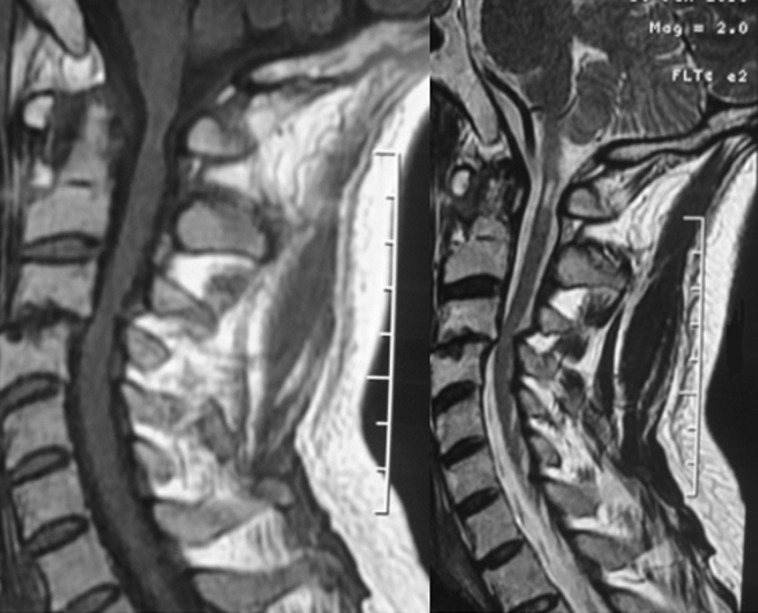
MRI appearance in T1 and T2 showing instability and signs of spinal cord pain
